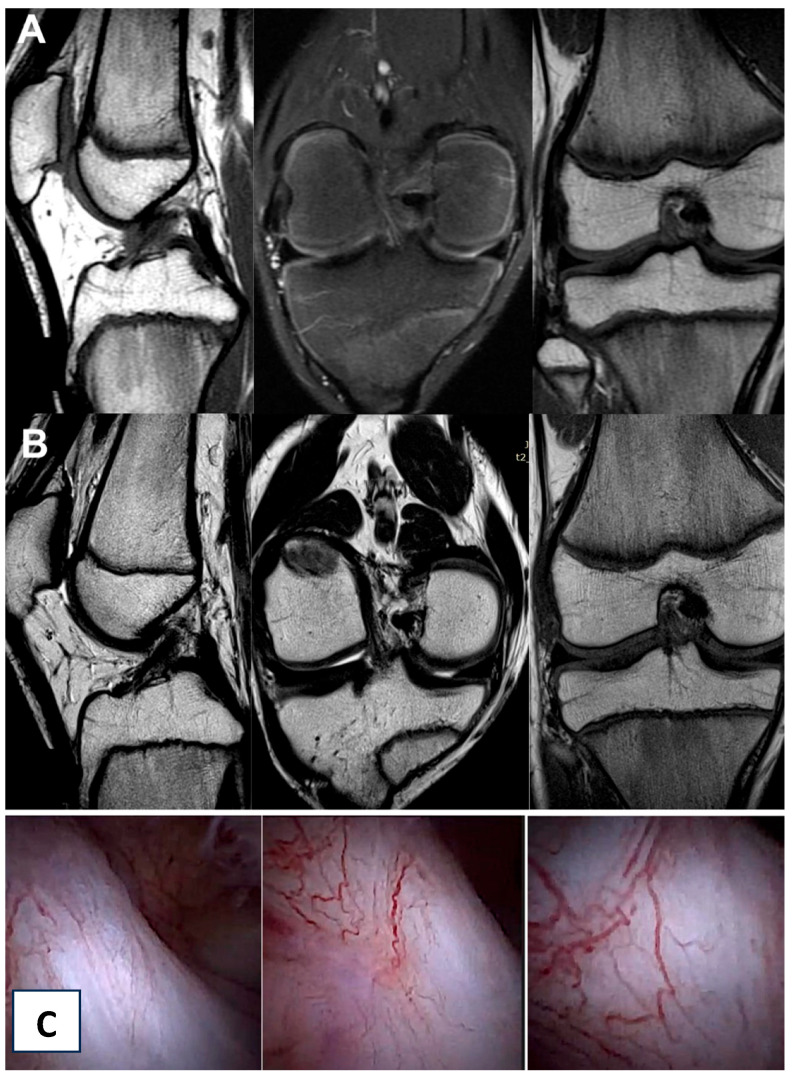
The same knee MRI scans of a patient with an anterior cruciate ligament tear before treatment (A) and corresponding MRI scans after nanosurgery treatment with RP-hCM injection (B). A nanoscopic view of the normal, healed ACL (C).
